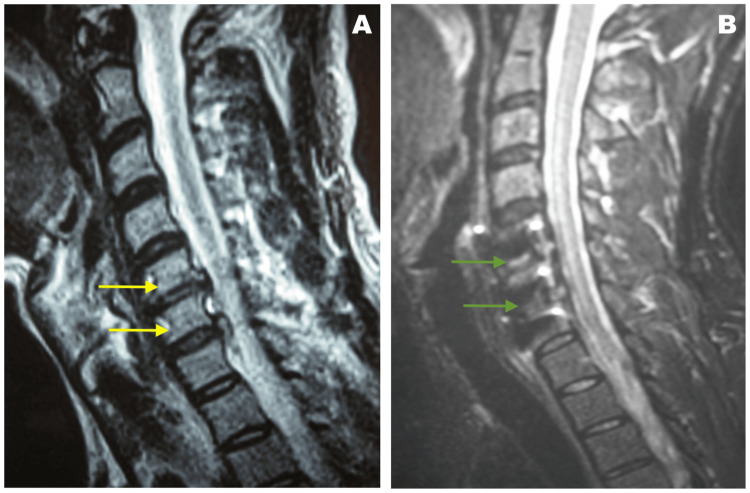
Magnetic resonance imaging findings. A: Preoperative imaging showing disc herniation (yellow arrows) at the C5-C6 and C6-C7 levels. B: Postoperative imaging after anterior decompression with the fusion of the affected levels (green arrows).
